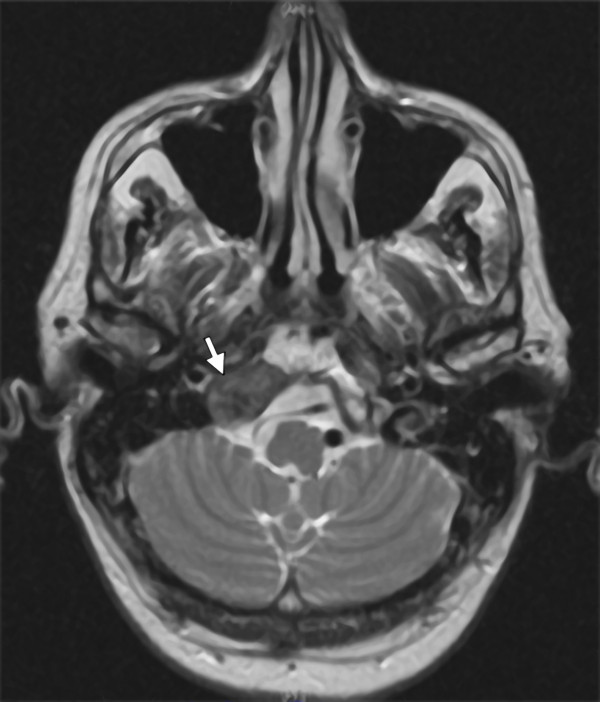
MRI scan with moderate degree of enhancement at the base of the skull, affecting the right occipital condyle and part of the right side of the basilar bone.
